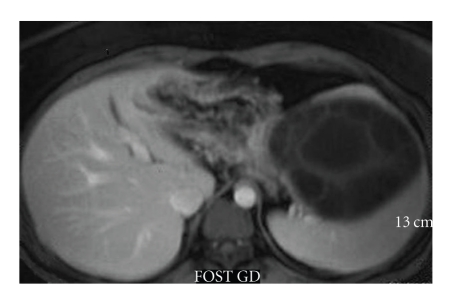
Axial contrast-enhanced T1-weighted MRI shows hypointense multivesicular splenic cyst showing no significant contrast enhancement, except some vague signal increase in the septal parts.
